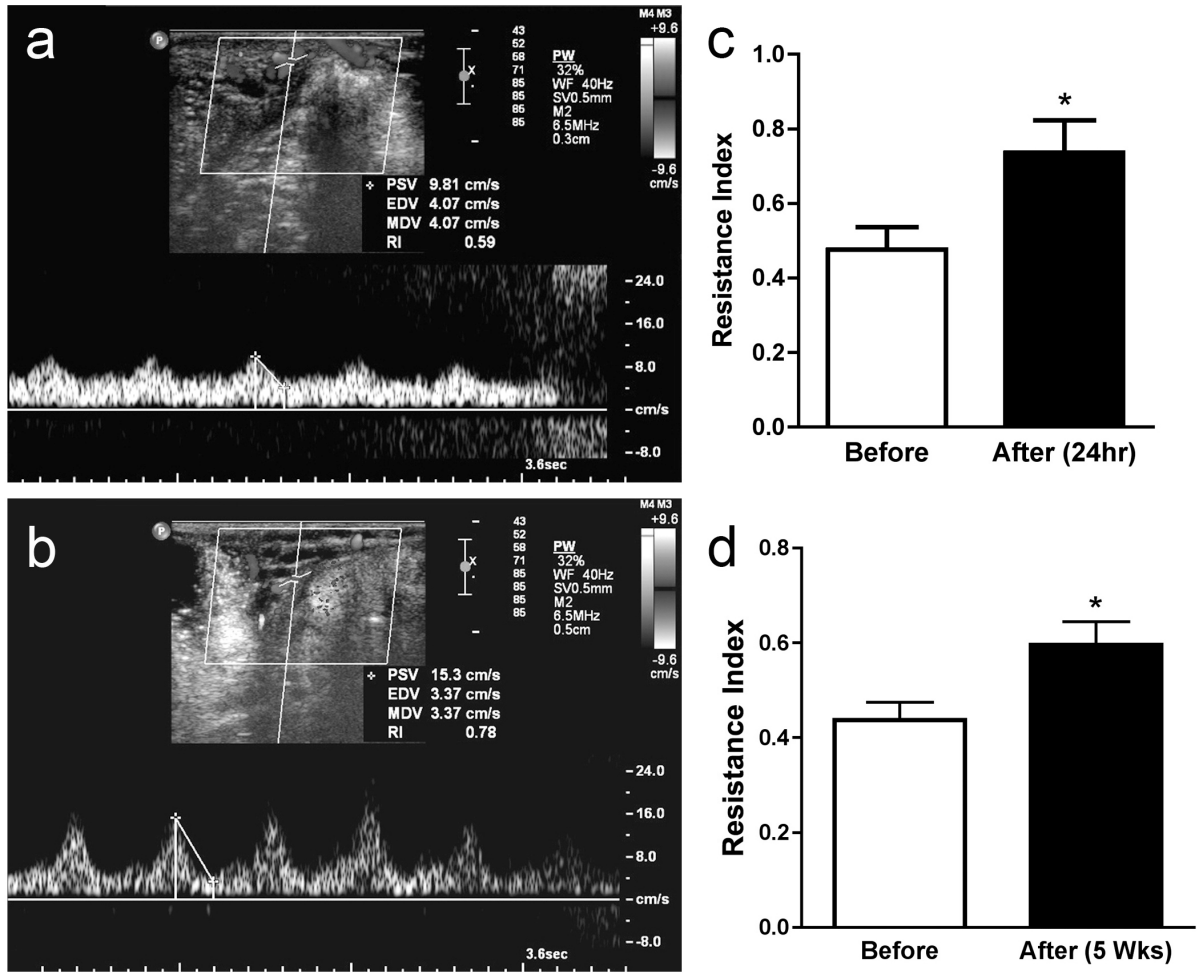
The effect of diathermy on the ovarian artery resistance index (RI). Representative RI scans with Colour Doppler signal wave quantification before (a) and 24 hours after ovarian diathermy (b). Quantified RI of the ovarian artery before and 24 hours (c) and 5 weeks (d) after diathermy. P*<0.05.
